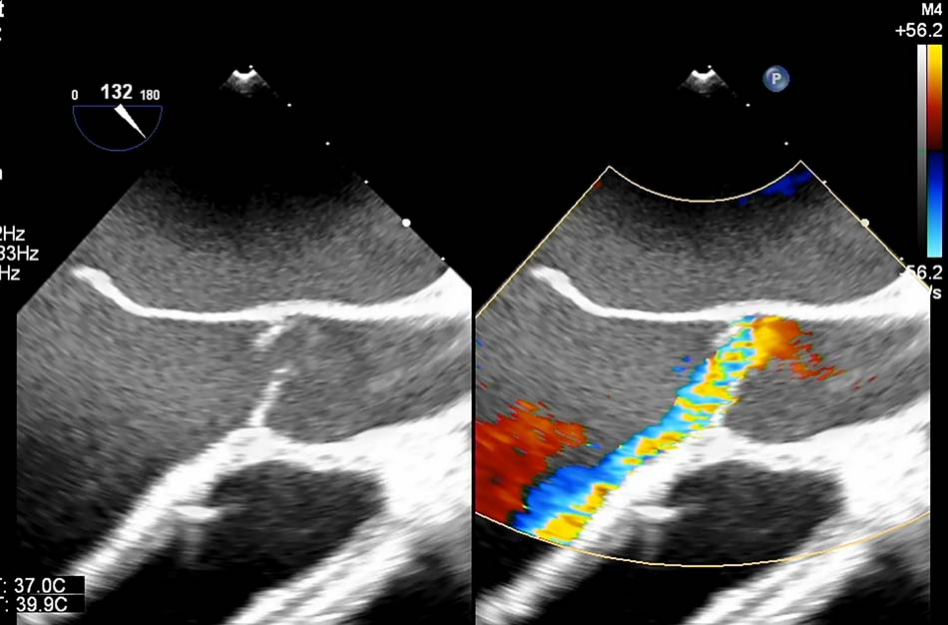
Transesophageal echocardiography showing moderate aortic insufficiency (AI) due to prolapse of noncoronary cusps after Impella removal.
